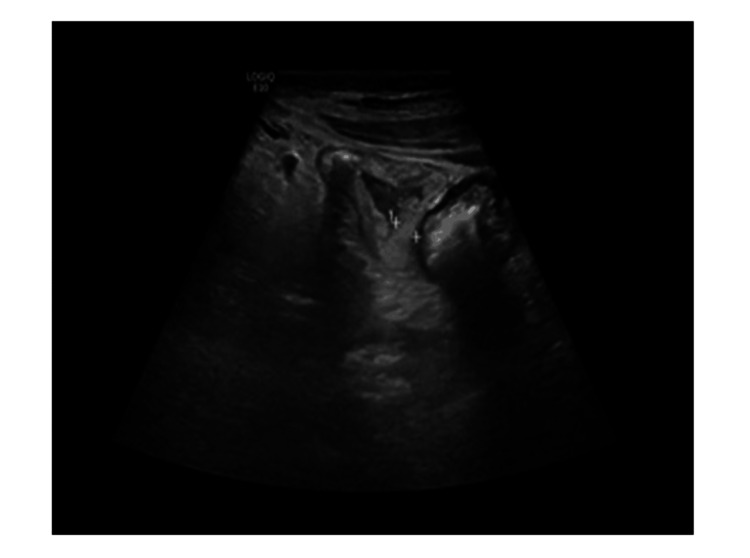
Ultrasound image of the small bowel.
